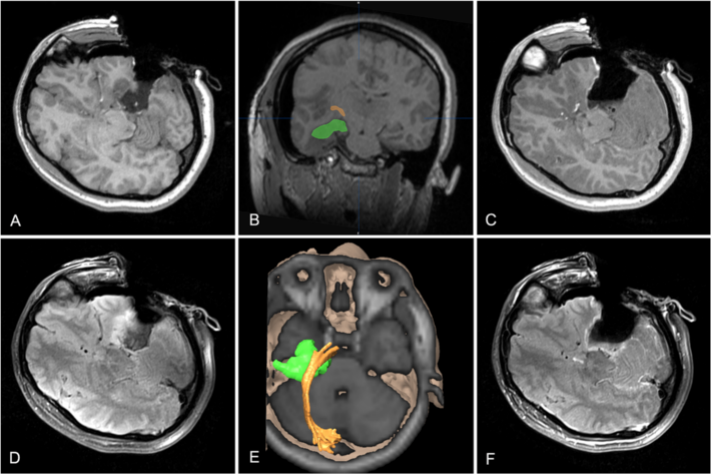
Intraoperative MR imaging. The same patient as in Fig. 1. The first iMRI scan demonstrated that the partial lesion prior to the cavity remained (a, d). After infusion of the intraoperative optic radiation tractography and the outlined residual tumor, the neuronavigation was updated (b, e). Final iMRI scan revealed that the tumor remnants had been removed (c, f)
